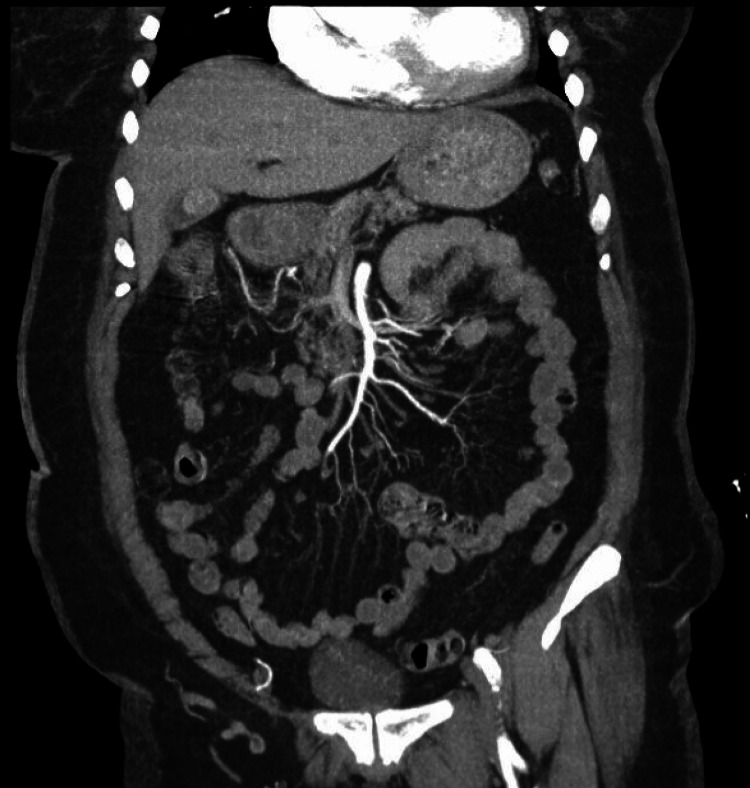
Contrast-enhanced CT angiogram of the abdomen and pelvis with contrast demonstrating a patent superior mesenteric artery (SMA) with no evidence of stenosis or occlusion.
